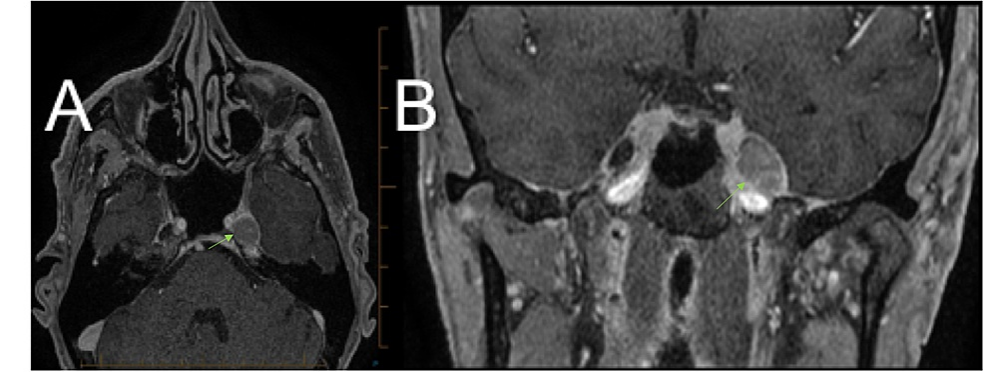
Magnetic resonance imaging brain (T1-weighted gadolinium-enhanced) taken on May 2017: (A) transverse and (B) coronal views demonstrating a left-sided lesion located in Meckel's cave with extension into the cavernous sinus.
